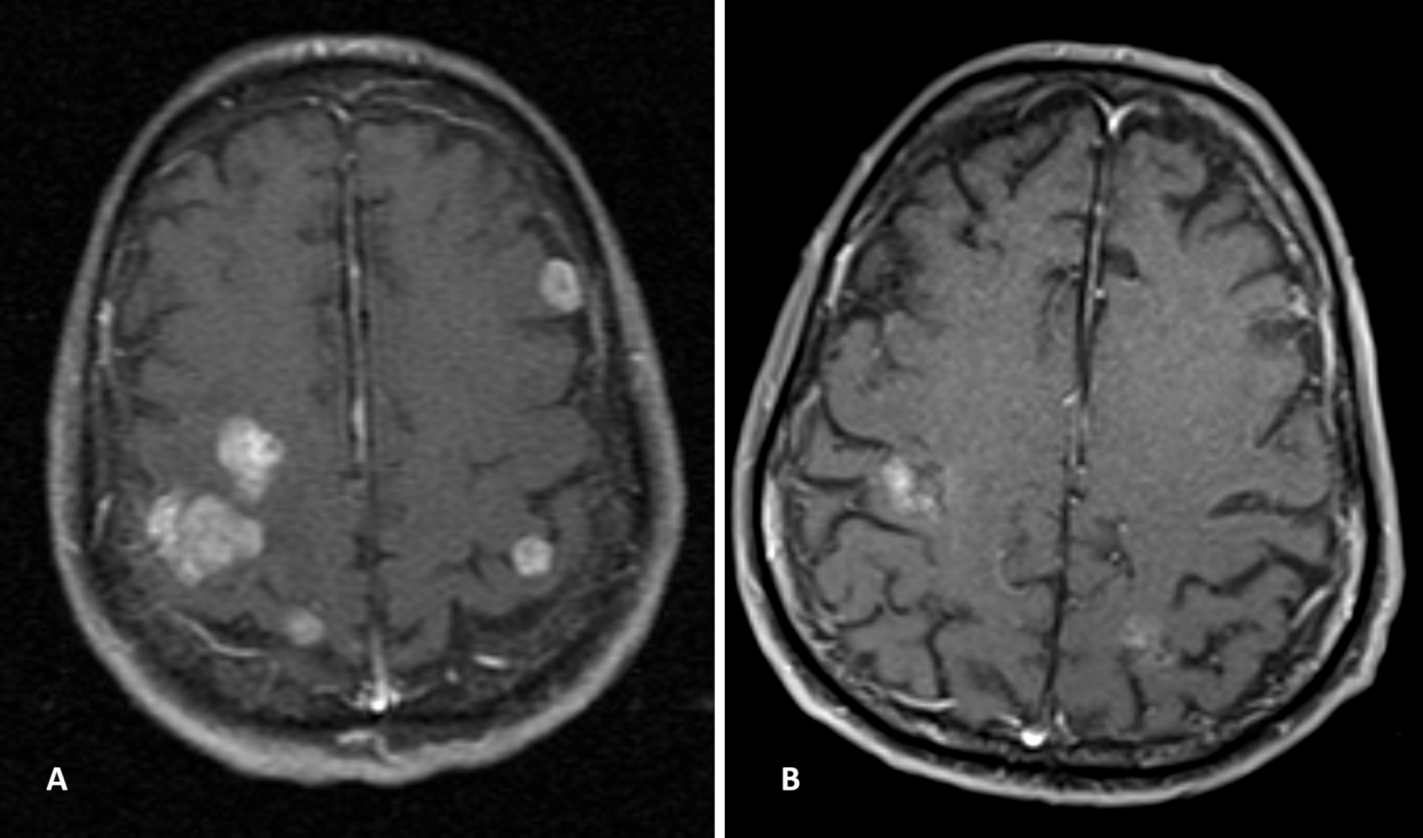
MRI scans of the brain in Patient 2 in February 2017 (A) and January 2019 (B).
